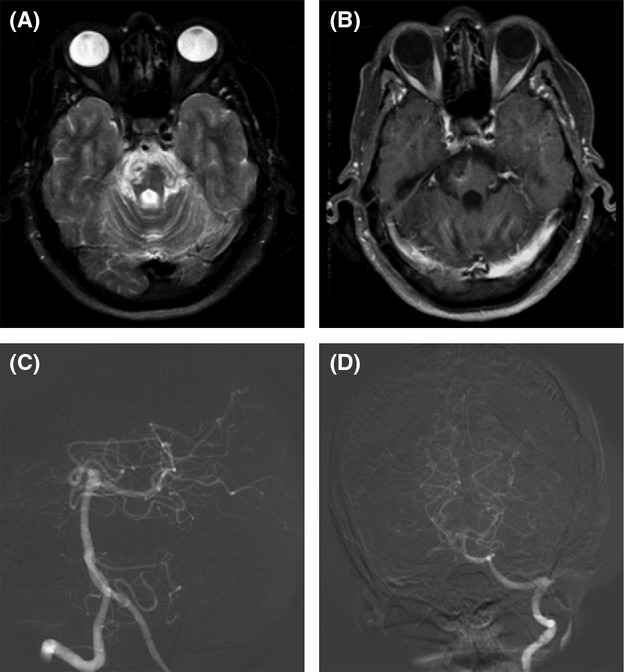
AVM located in right pons after gamma knife treatment. (A) Axial T2-weighted MRI brain. (B) Axial T1-weighted MRI brain with contrast. (C) Digital subtraction arteriogram, vertebral artery injection, lateral view, arterial phase. (D) Digital subtraction arteriogram, vertebral artery injection, anterior–posterior view, arterial phase. AVM, arteriovenous malformations; MRI, magnetic resonance imaging.
